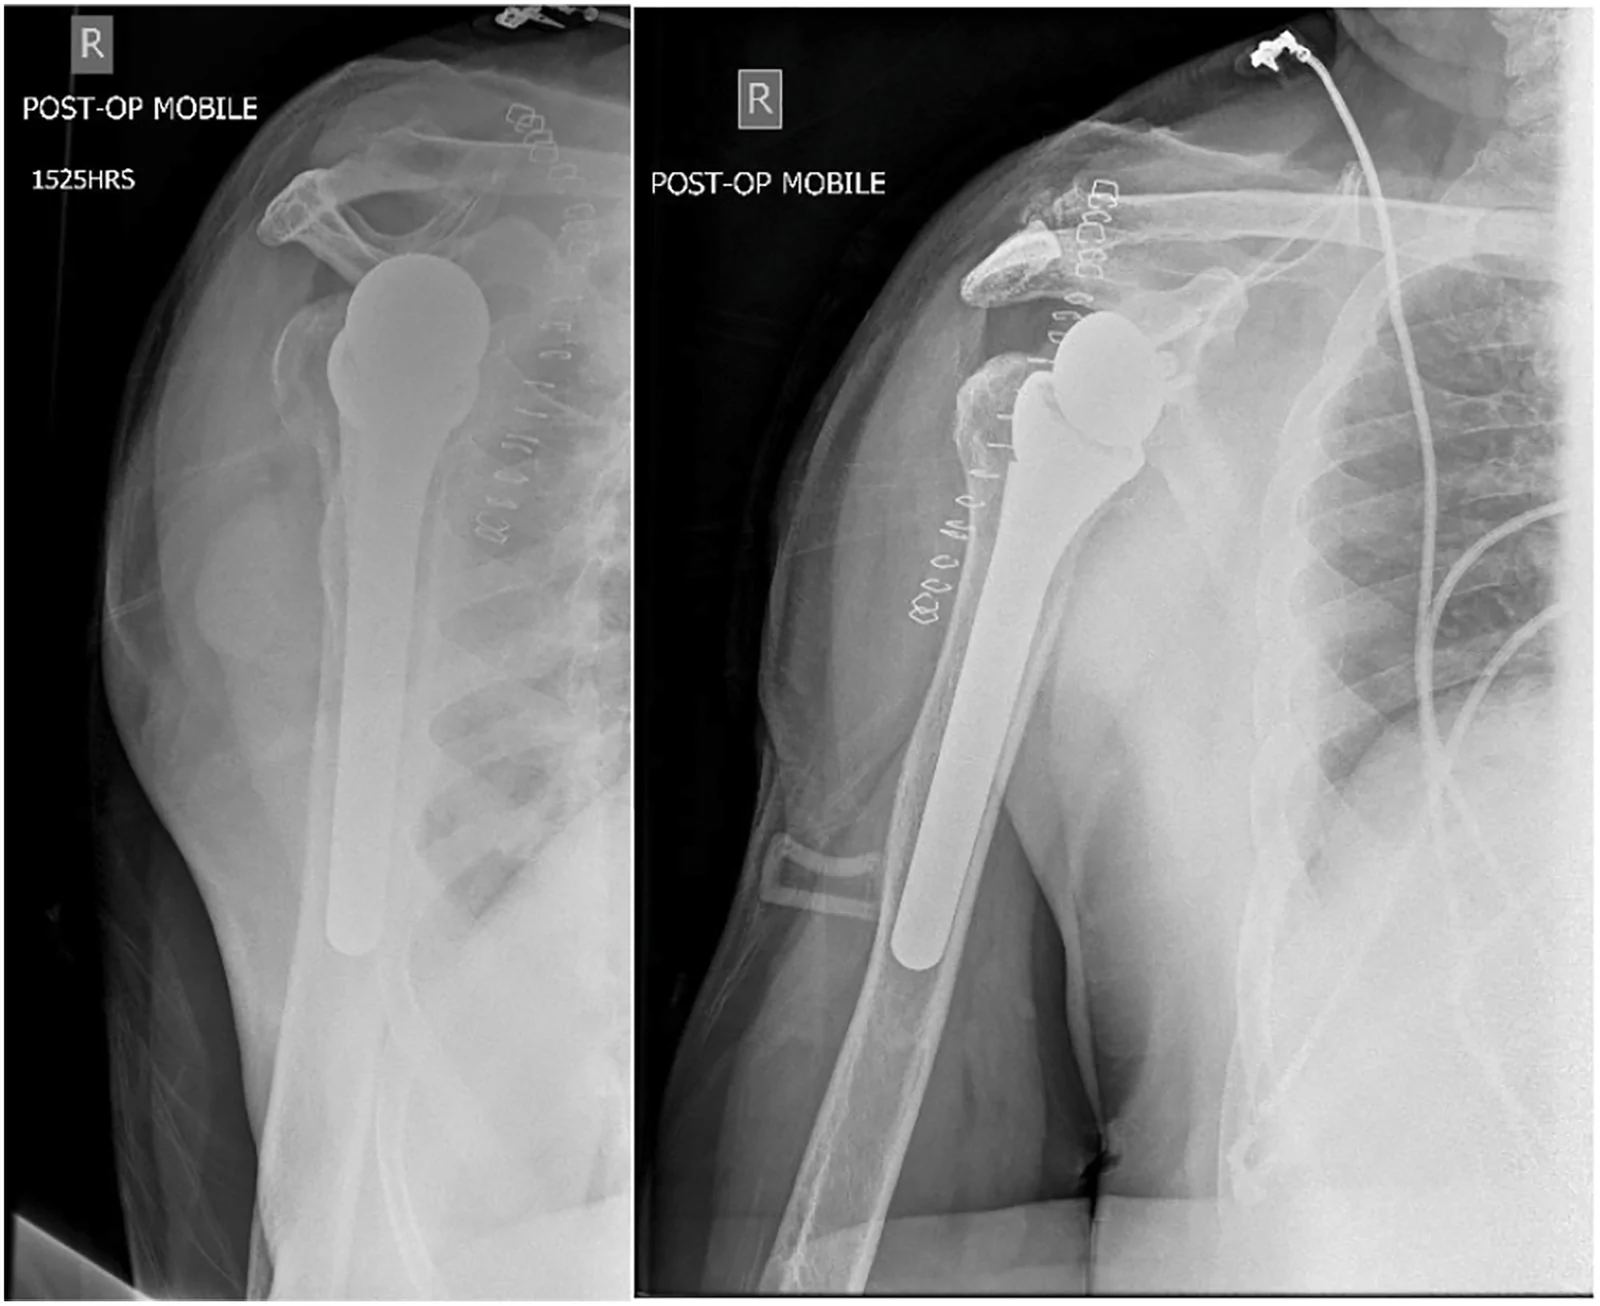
Case 1 immediate post-operative x-rays.
